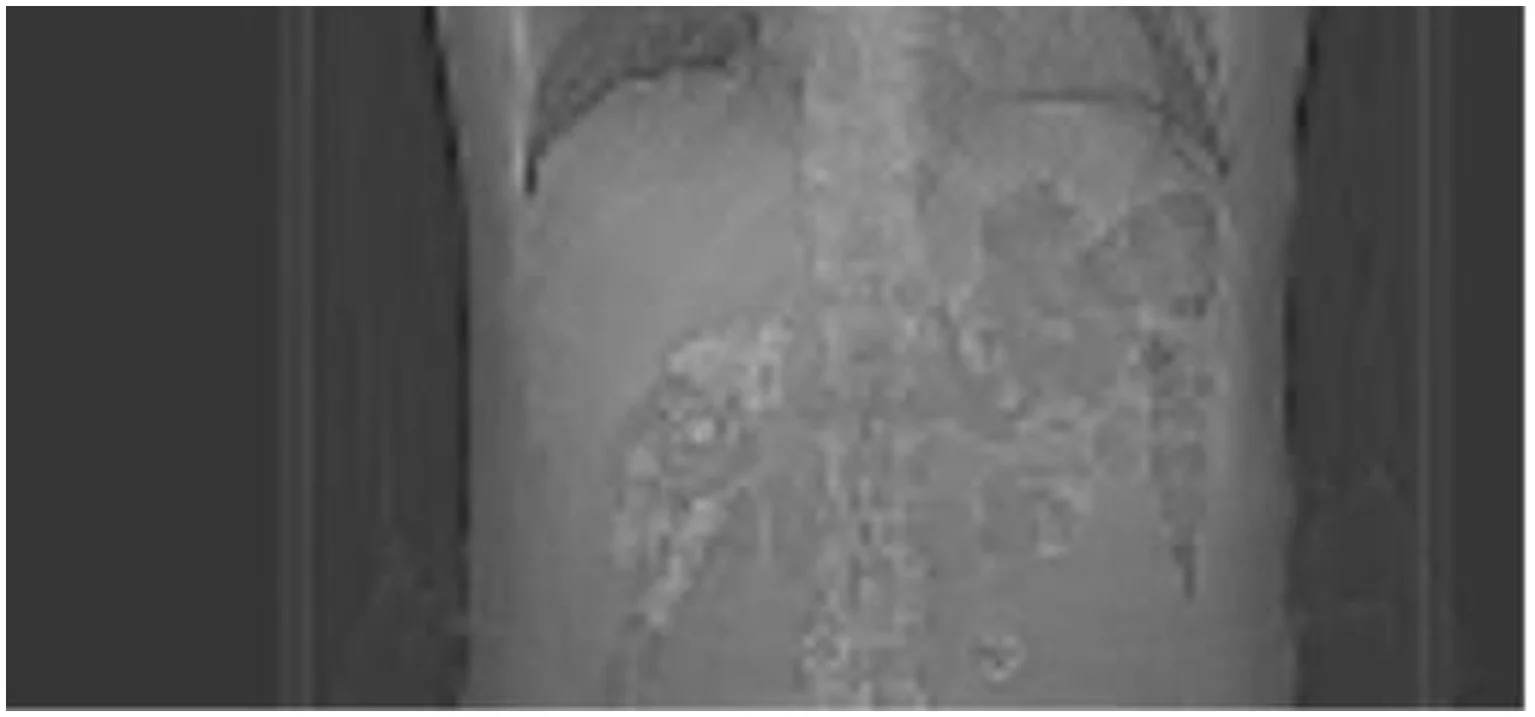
The low-dose non contrast CT scan of upper abdomen region showing sever bilateral nephrocalcinosis (Patient 1).
